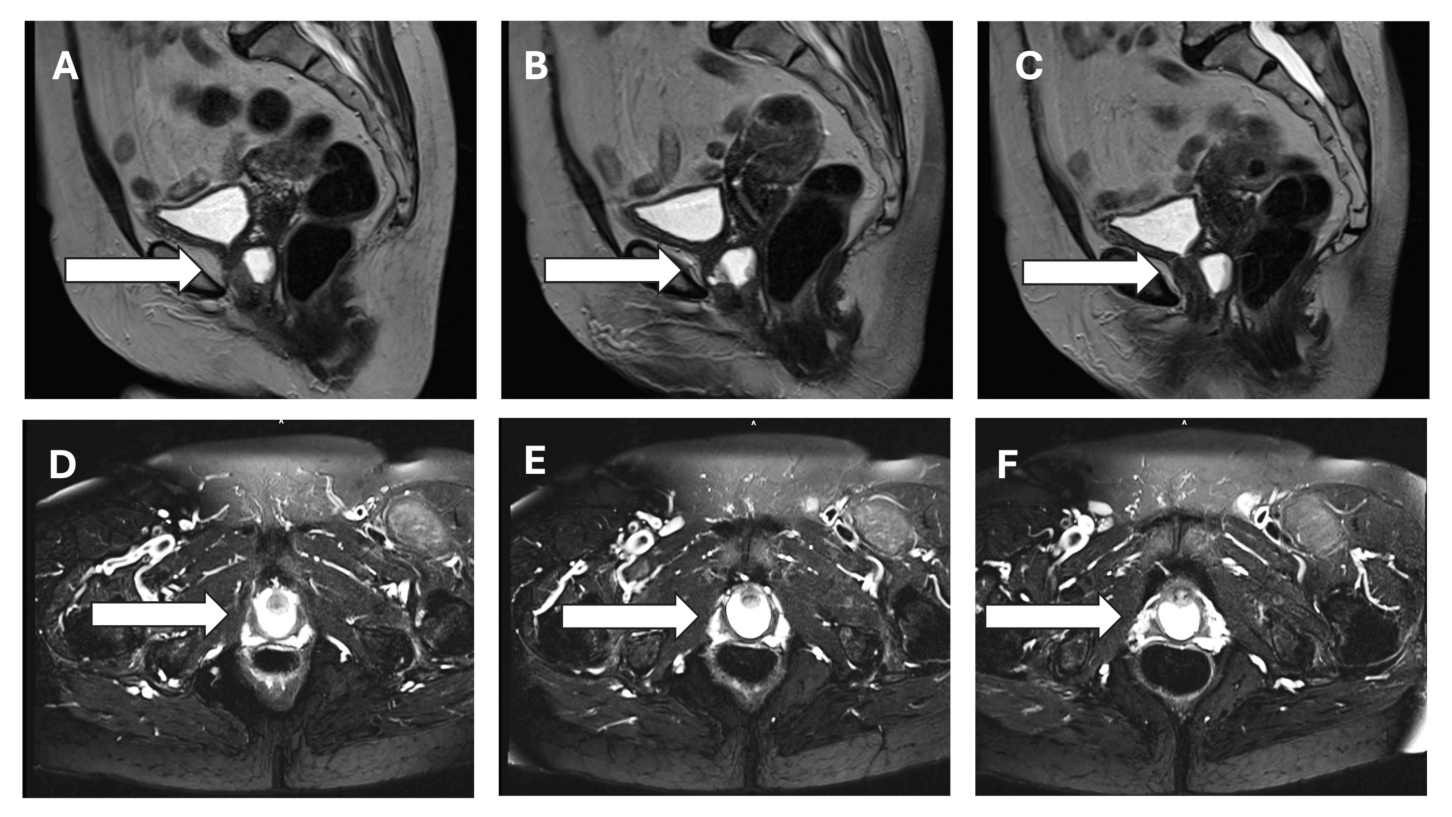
Sagittal (A-C) and axial (D-F) views of the urethral diverticulum T2-weighted magnetic resonance imaging of the urethra after 8 mL of intravenous contrast was injected. A 2.0 × 2.5 × 2.0 cm periurethral diverticulum (white arrow) with a small fluid level and mild thin internal stranding was noted. The white arrows point to the filling of the urethral diverticulum with contrast at progressive time points during the scan. A mass effect was noted from the diverticulum anteriorly.
